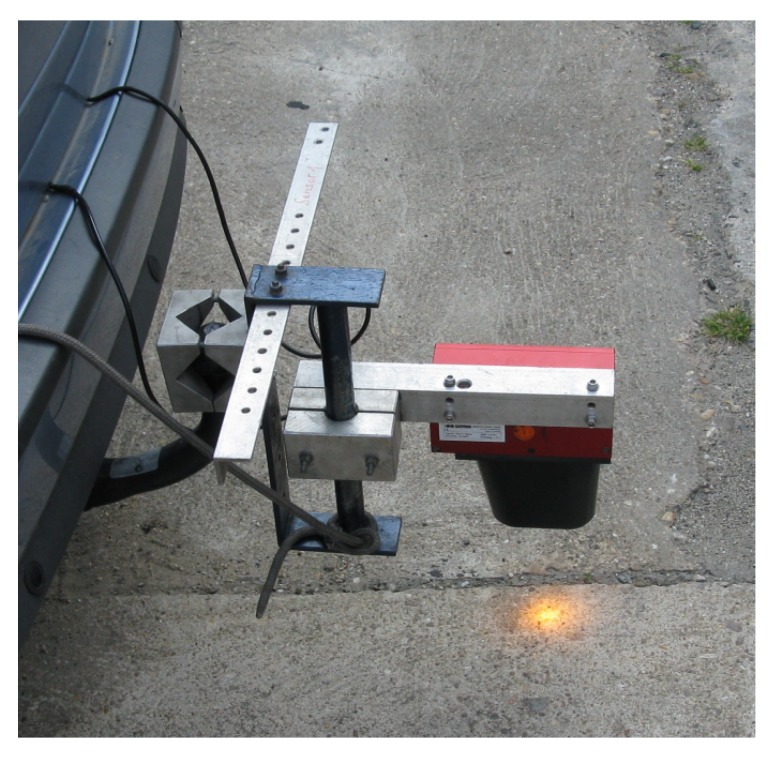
Laser Doppler velocimeter used to generate reference data for the validation of the trajectory reconstruction.
